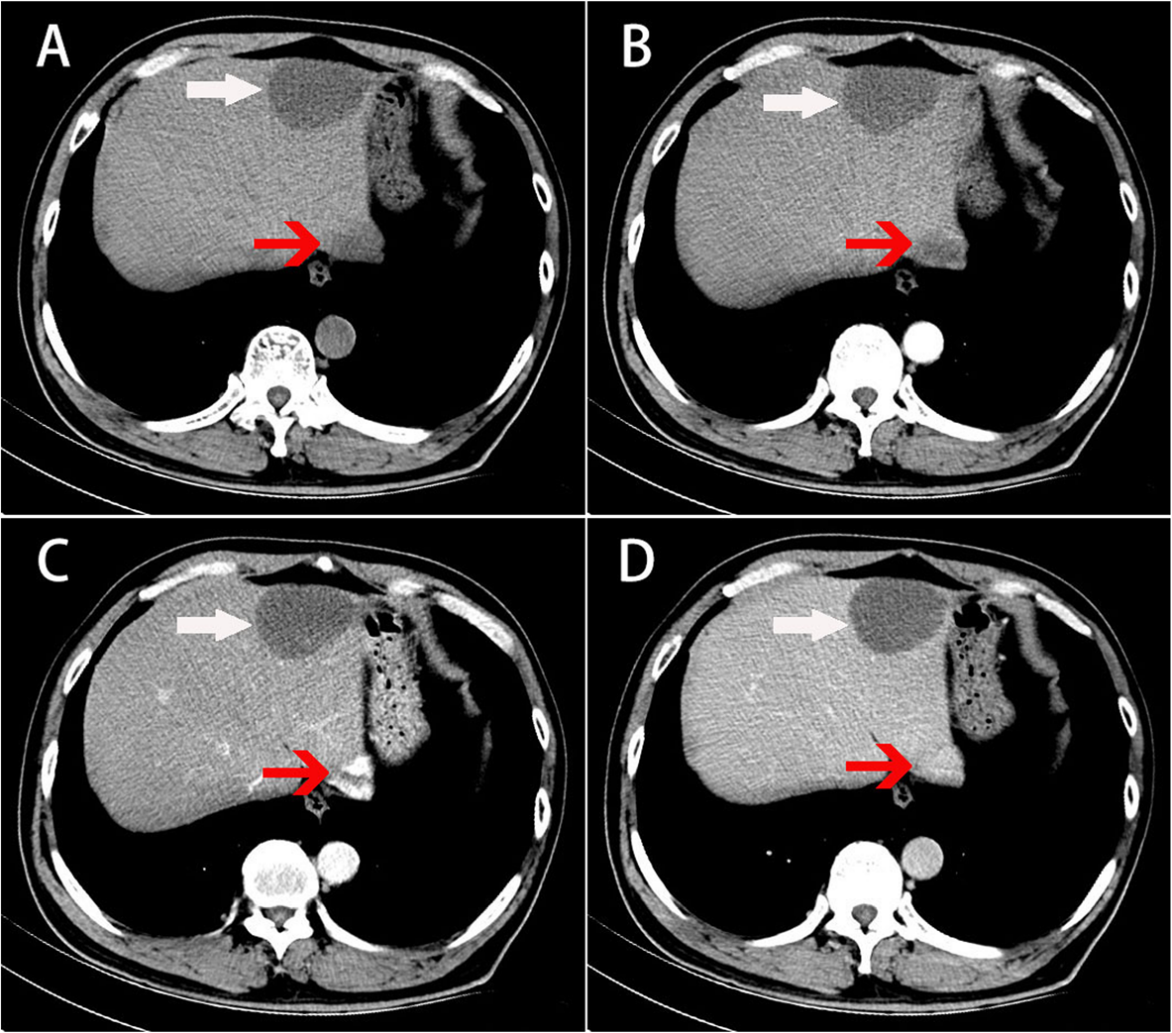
Enhanced CT scans. a Plain scan, b arterial stage, c portal vein stage, d and delayed stage. A patchy low-density shadow without significant enhancement can be seen in the liver (white arrow). Cavernous hemangioma (red arrow)
